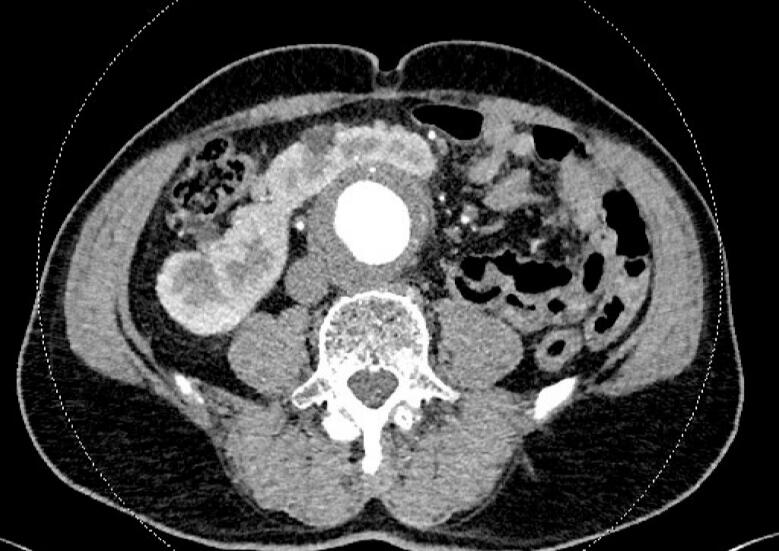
CT morphological position HEN and aorta.
